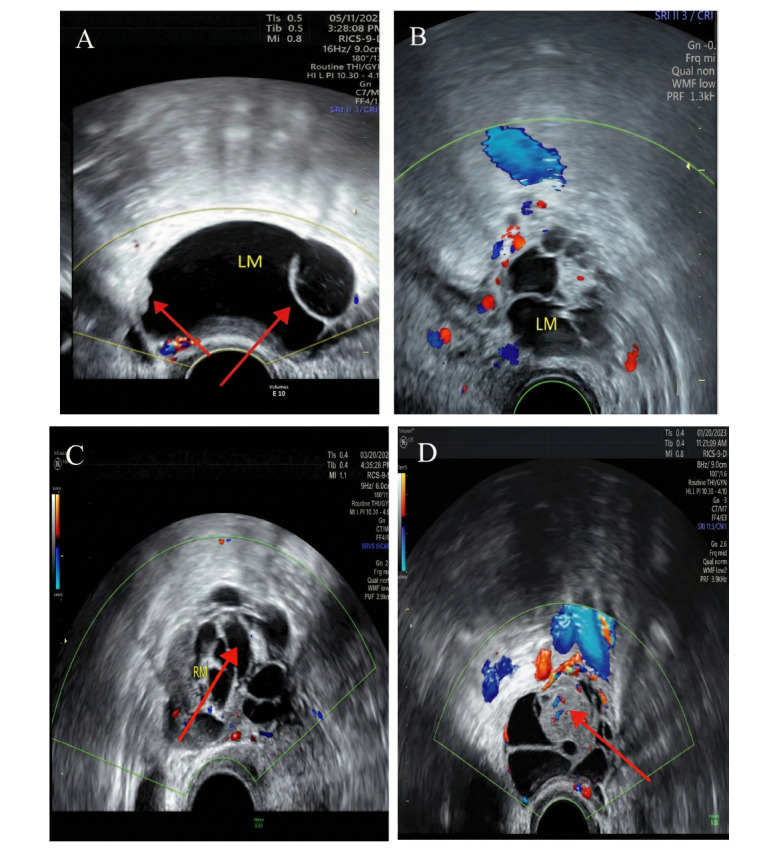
Ultrasonography of blood flow grading and internal septa (arrows) of ovarian mass. (A) Struma ovarii with blood flow grade 0. (B) Cystic mass with the blood flow grade I in the ovary. (C) Ovarian cystic mass with multi-septum and blood flow grade II. (D) Struma ovarii with cystic structures of various sizes in the left ovary, containing glial elements and raising the suspicion of goiter of the ovary. The interior is primarily cystic, and the blood flow grade is grade III.
